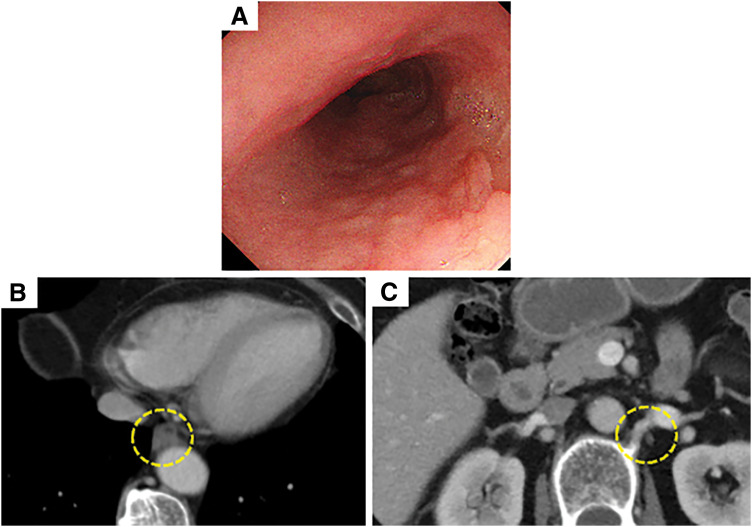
Imaging findings after 3 cycles of pembrolizumab plus FP therapy. (A) The tumor in the midthoracic esophagus showing size reduction. (B) CT shows improvement in the wall thickening of the midthoracic esophagus (yellow circle). The longest diameter of the primary esophageal lesion decreased from 21 to 12 mm, corresponding to a 42.9% reduction. The overall treatment response was classified as a partial response according to RECIST criteria. (C) The para-aortic lymph nodes are decreased in size (yellow circle). FP, cisplatin and 5-fluorouracil
